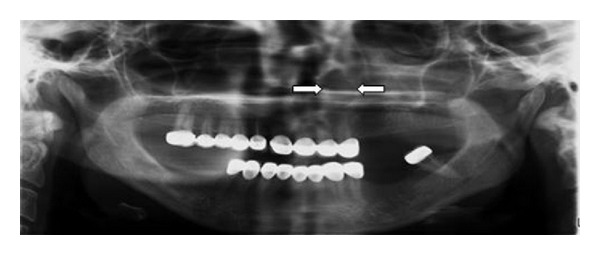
Bone alterations on panoramic radiograph.
